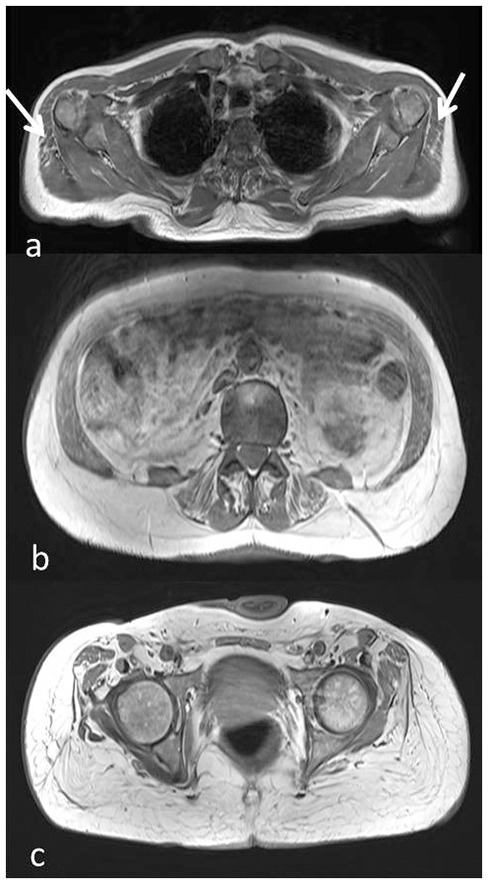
Muscle MRI axial T1-weighted images at the level of the shoulder girdle (a), lumbar muscles (b), and pelvic girdle (c), showing minimal adipose involvement of the deltoid muscles (a, arrow), discrete fatty substitution at the level of the abdominal and lumbar muscles (b), while gluteus maximus and medium are completely substituted (c).
